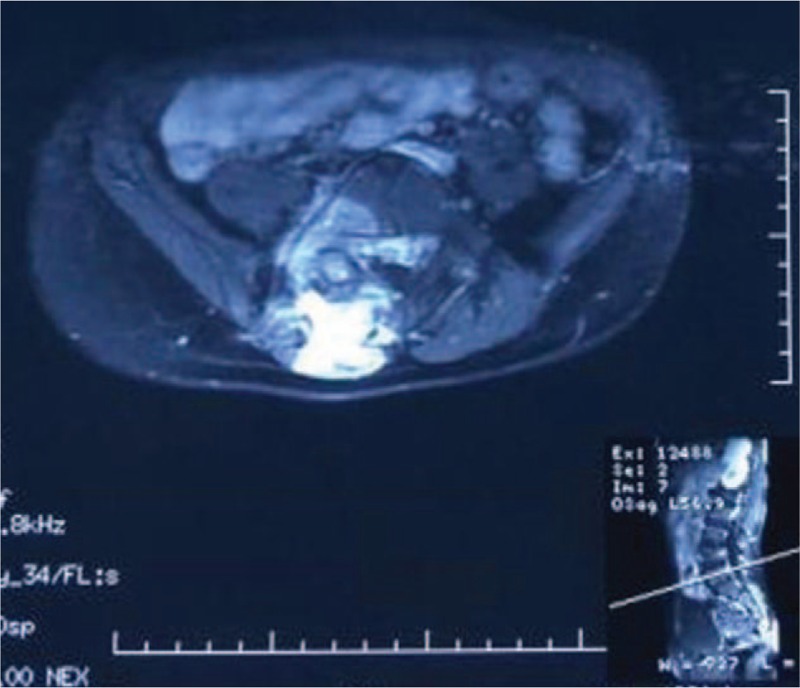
Enhanced MRI. MRI = magnetic resonance imaging.
